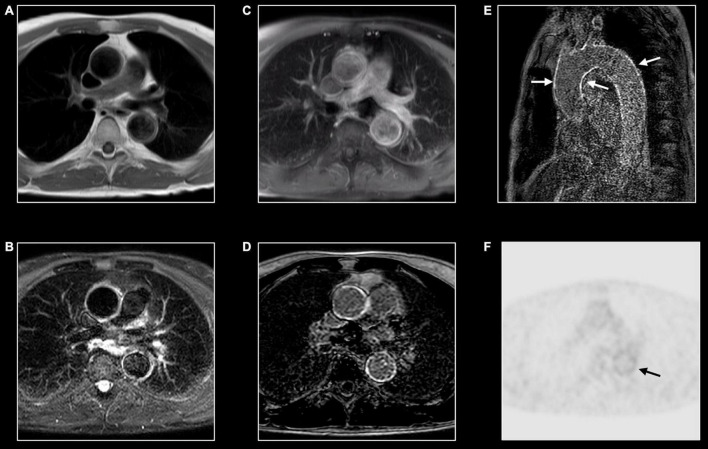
Immunoglobin G4-mediated aortitis in 57-year-old man. T1w imaging (A) shows mild dilatation of the proximal descending aorta with mild wall thickening. Hyperintense appearance of both ascending and descending aorta at T2w imaging (B) with contrast enhancement at post-contrast T1w imaging (C) and LGE imaging (D). A long-axis view through the thoracic aorta nicely shows the diffuse wall enhancement [arrows, (E)]. FDG-PET shows mild to moderate tracer uptake [arrow, (F)] in the descending aorta [arrow, (F)].
